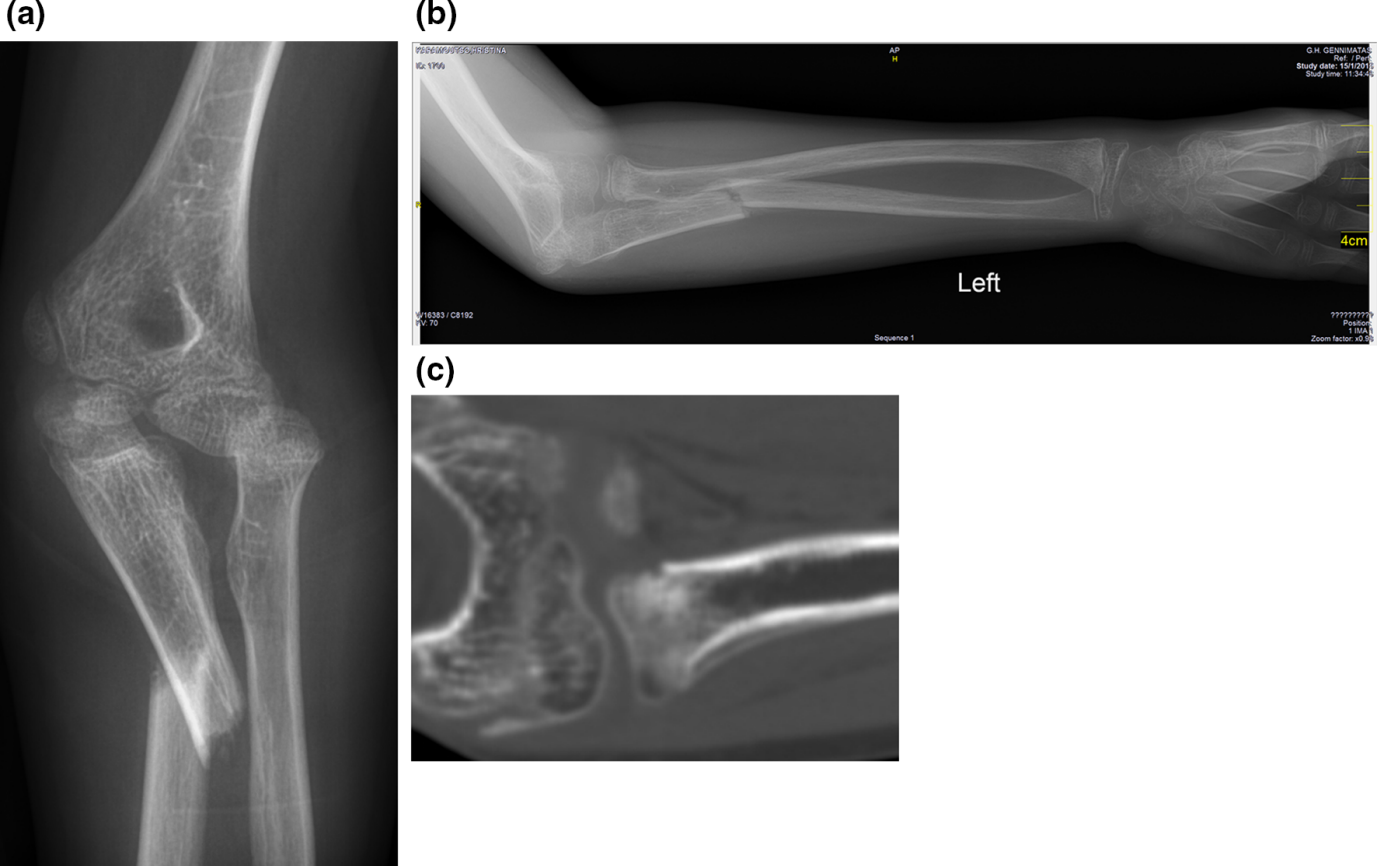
An 11-year-old girl with a lateral type Monteggia injury (a) associated with fractures of the distal part of the radius and the ulna. Radiographs at 5 weeks showed periosteal healing of the proximal radial metaphysis (b). Computed tomography (CT) indicated an additional fracture of the proximal radial metaphysis (c). A type V segmental injury of the radius and a type III segmental injury of the ulna were diagnosed
